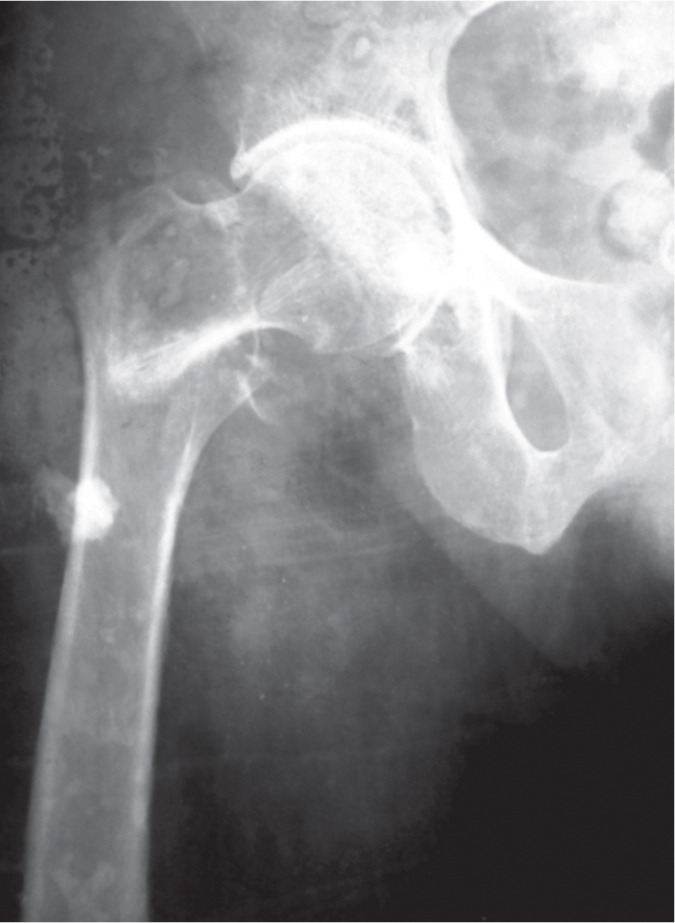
Preoperative Radiograph AP.
